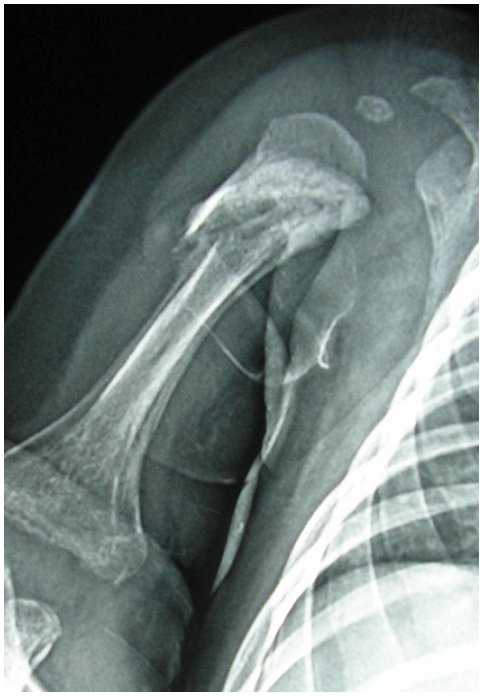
Radiographie montrant une fracture de l'humérus droit chez un 2 mois et 22 jours pris en charge pour syphilis congénitale révélée par une fracture spontanée
